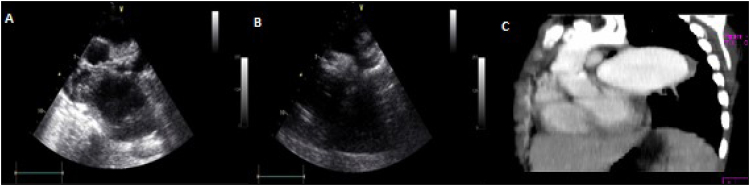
A and B. Echocardiogram in suprasternal view appreciating aortic arch with coarctation and saccular image in descending aorta that generates compression of the left pulmonary artery. C. Angiotomography: Aortic aneurysm after coarctation.
